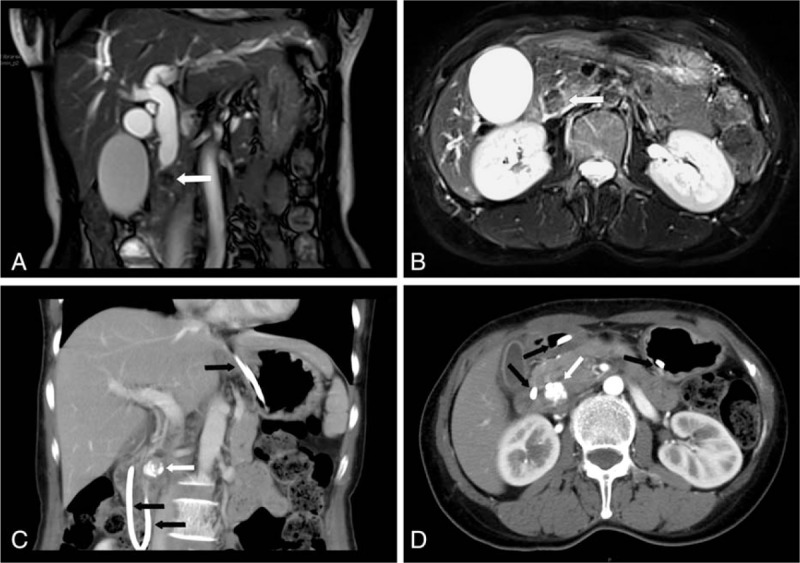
Abdominal MRI (A, B): nodular low-signal shadow (white arrow) was seen at the lower segment of common bile duct. Abdominal contrast-enhanced CT (C, D): nodular high-intensity shadow (white arrow) was observed in the upper duodenum. (Black arrow indicating nasobiliary drainage). CT = computed tomography, MRI = magnetic resonance imaging.
